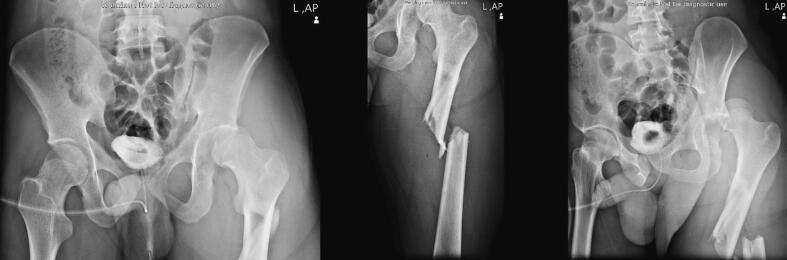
Plain anteroposterior radiography of the pelvis and femur shows a comminuted fracture of the femoral subtrochanteric region and a posteriorly dislocated hip.
